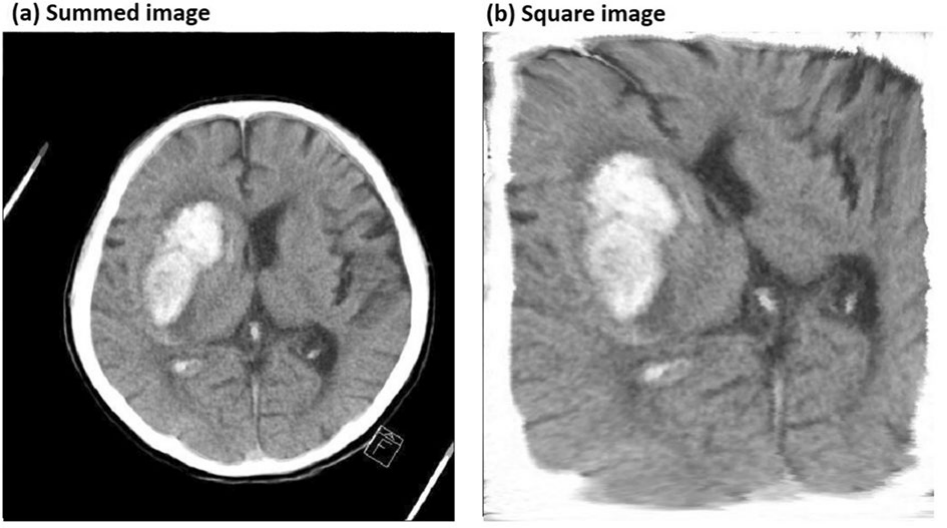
Example of CT image processing for preparing image data. (a) Summed image of a subdivision for a case. (b) Square image expanded to fit in a square.
